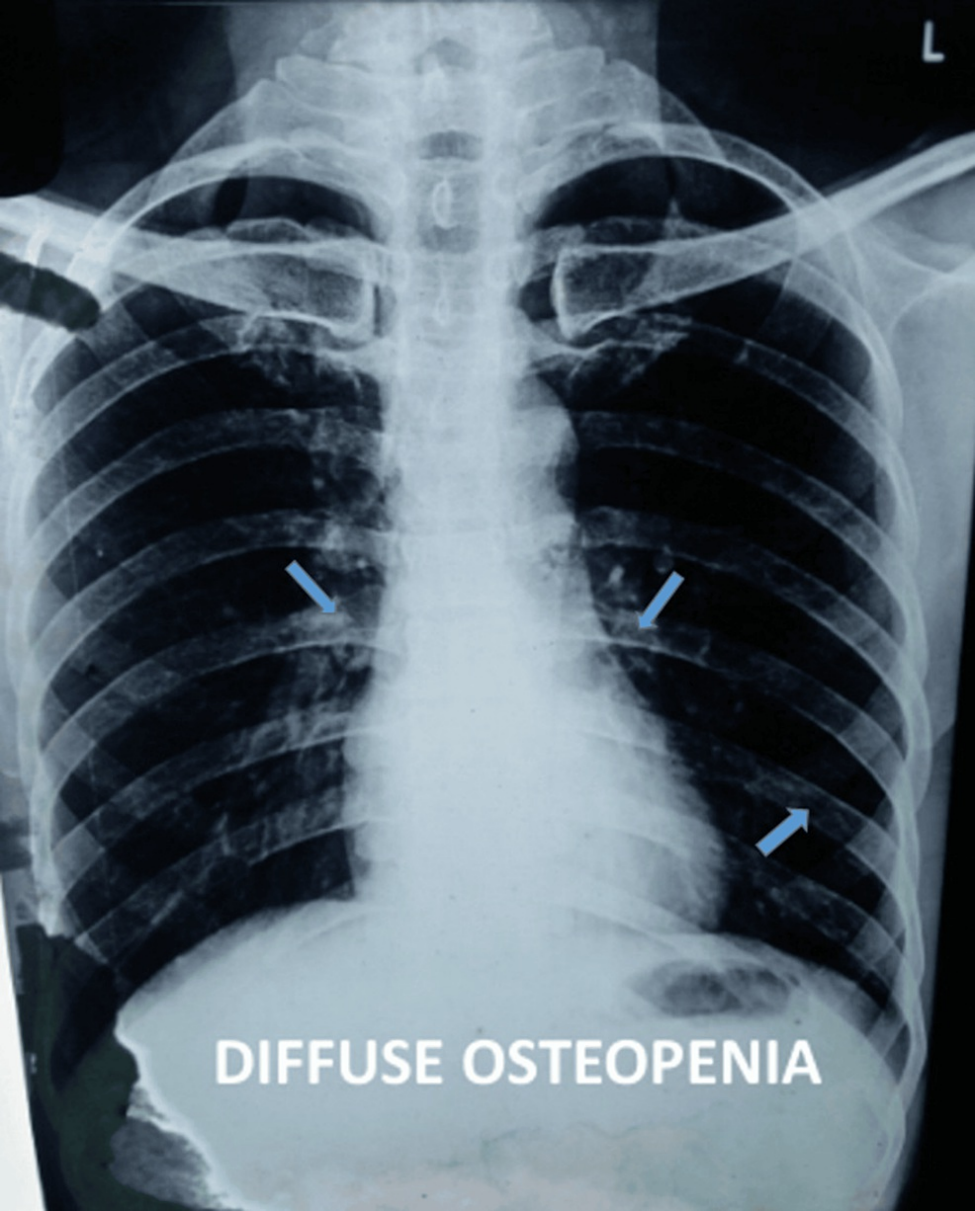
PA view of chest X-ray displaying areas of diffuse osteopenia (blue arrow). PA: posteroanterior
